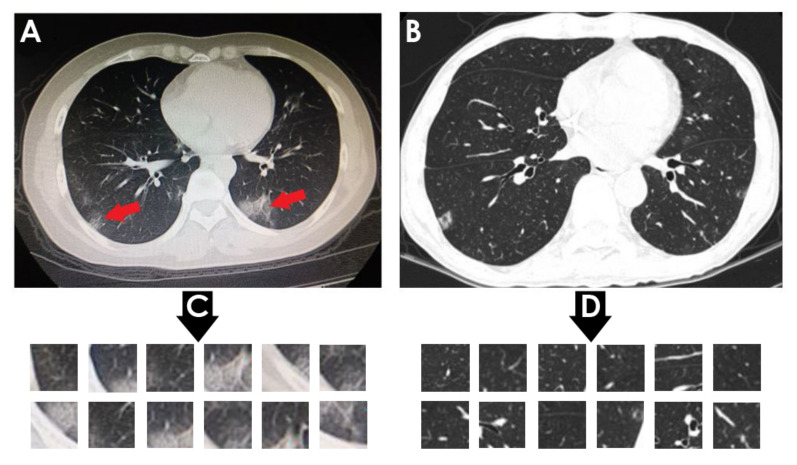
(A) Shows a CT of the lungs of COVID-19 (+) patient, in which there are multiple patchy ground-glass opacities in bilateral subpleural areas indicated by red arrows. (B) Represents a CT of the lungs of a COVID-19 (−) patient with normal controls. (C) Depicts infected patch samples. (D) Reflects non-infected patch samples. COVID-CT dataset [22] is the source for these images.
